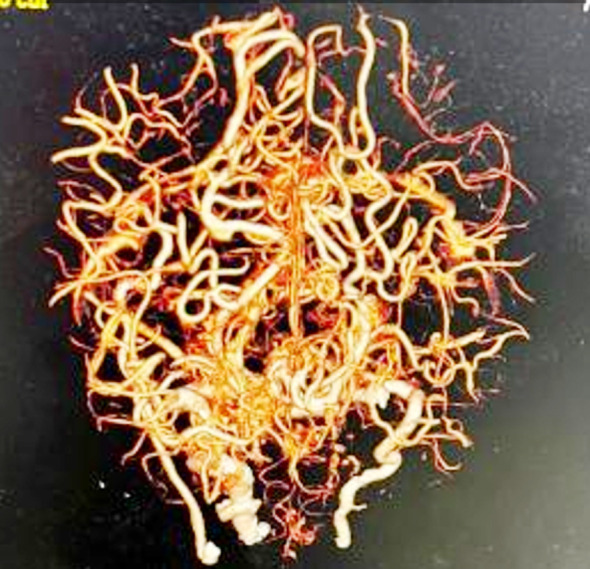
CT angiogram of brain showing coiled network of vessels at the base of brain giving puff of smoke appearance “Moya Moya”. (Picture courtesy: Dr. M. Farid, Dept. of Radiology, SIUT)
